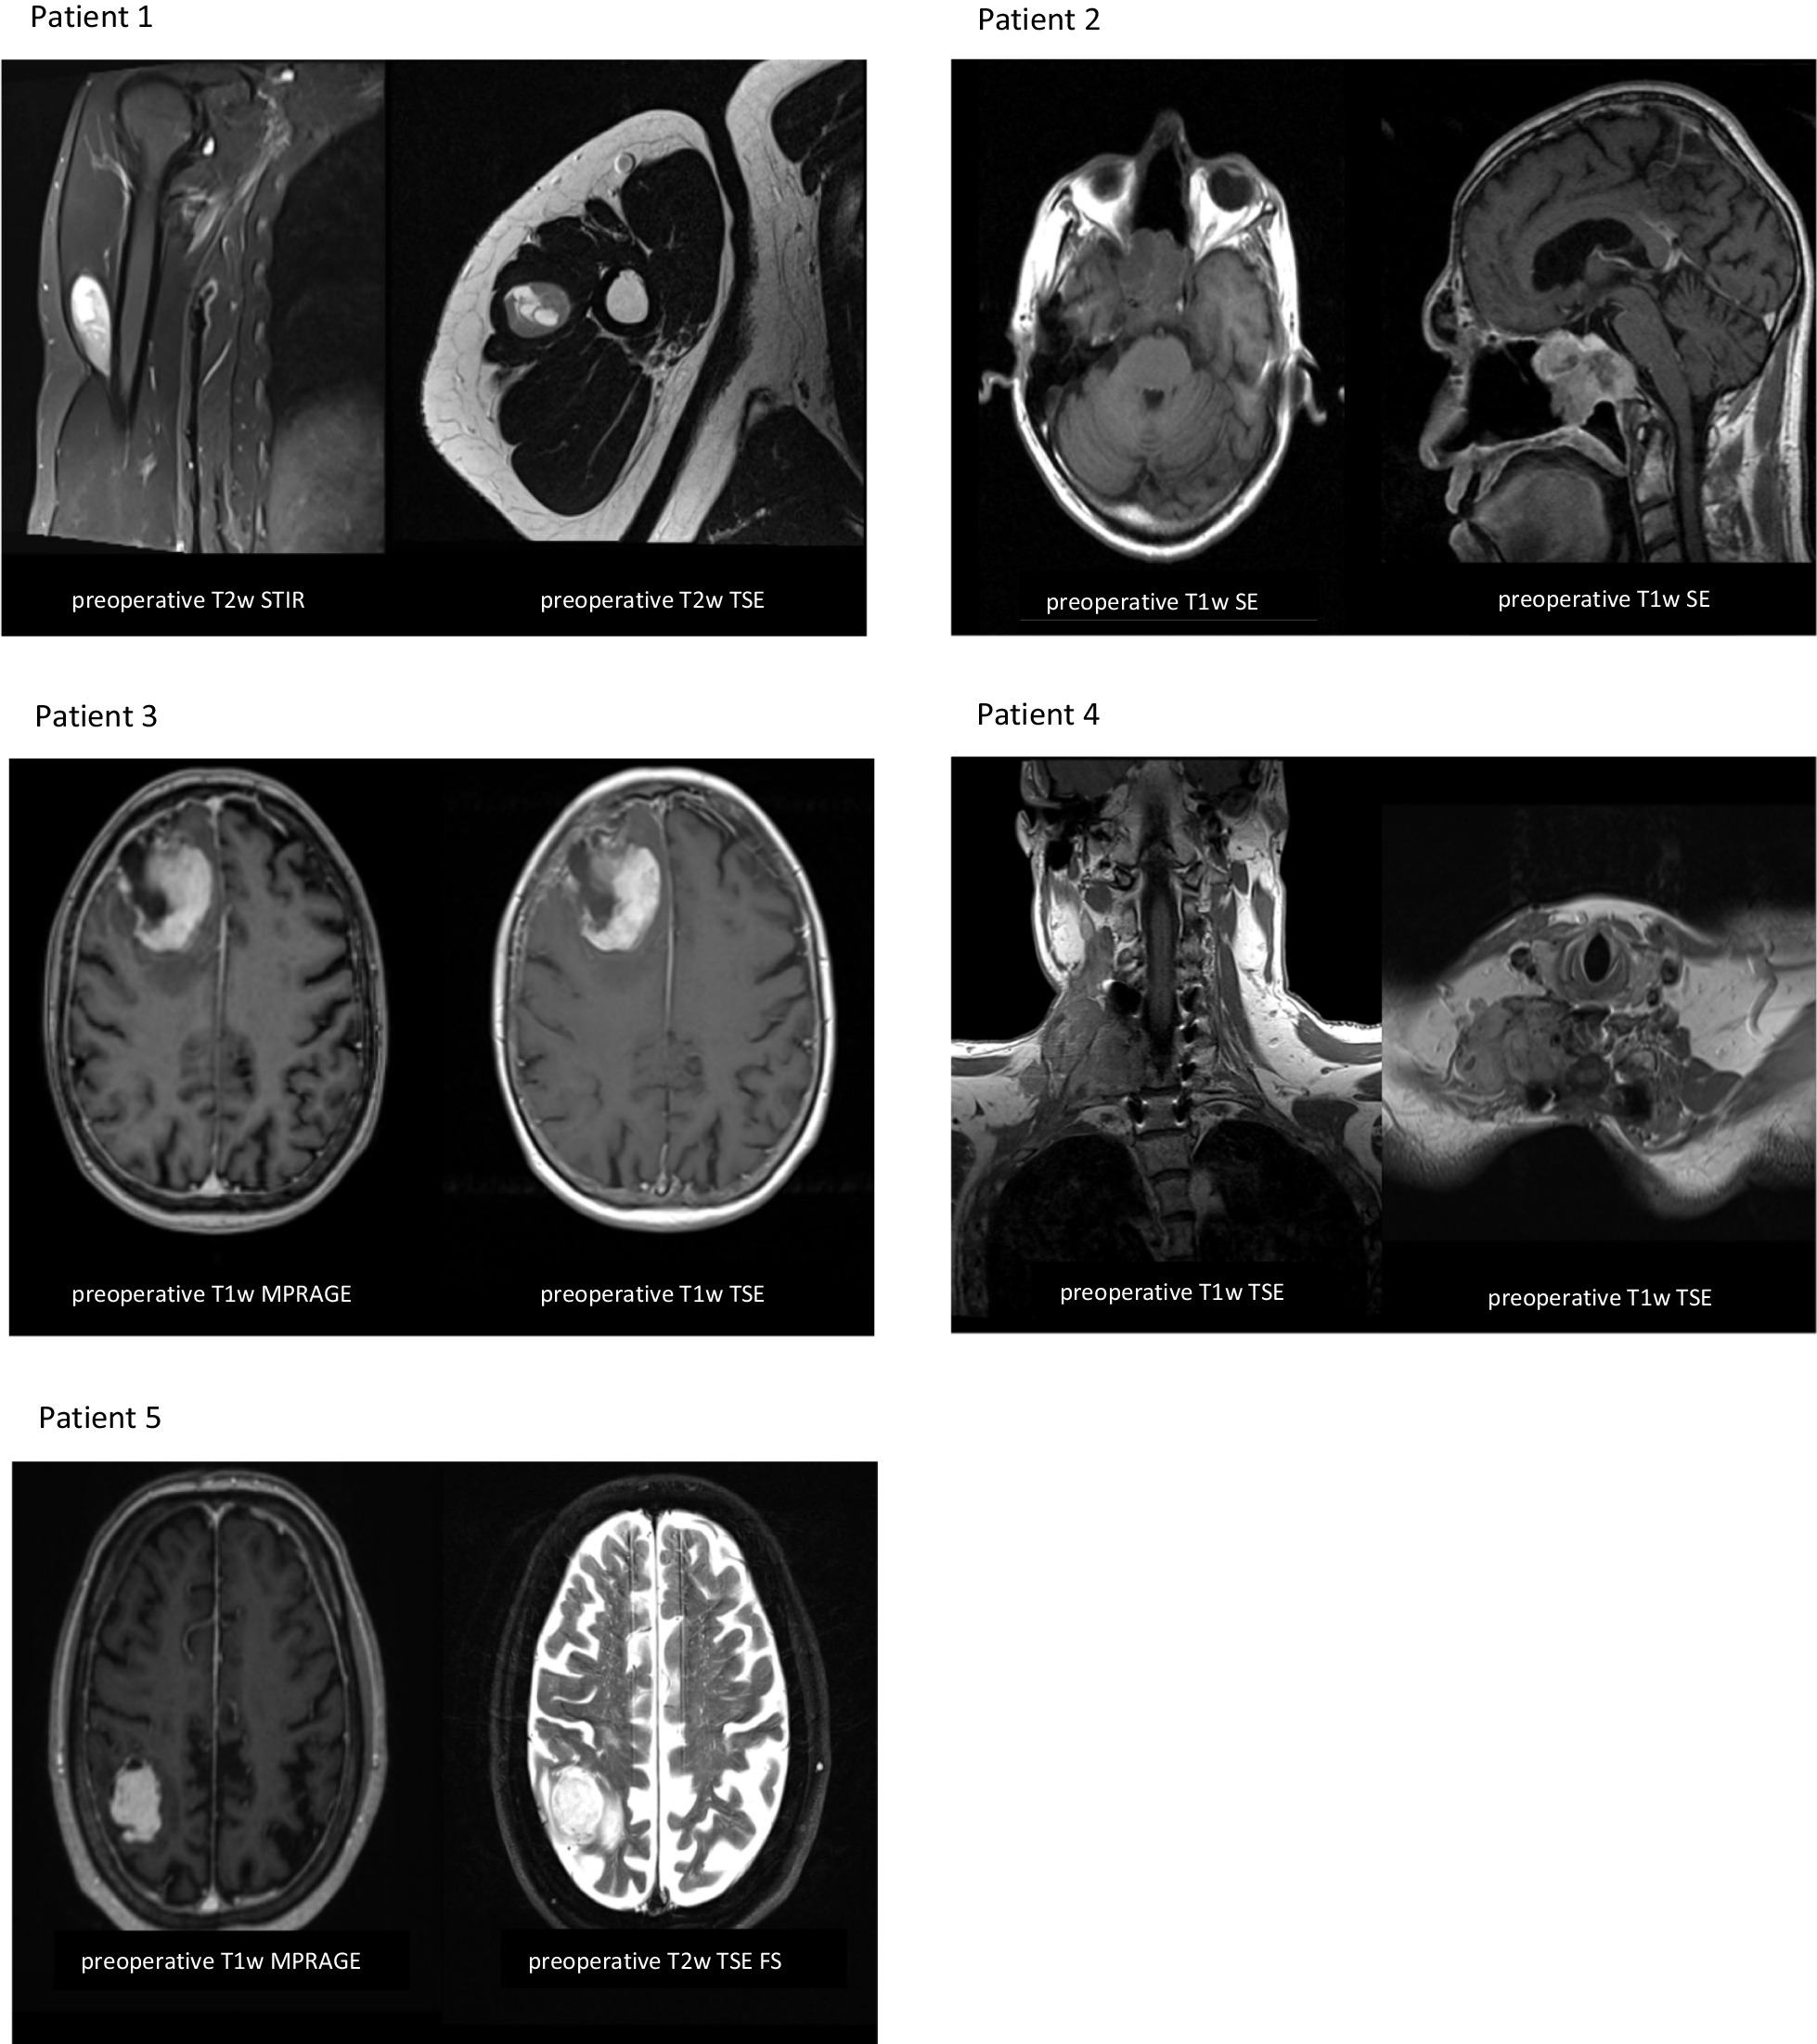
Preoperative MRI images. Representative MRI at the time of radiographic diagnosis and before treatment. *FS* fat saturation, *MPRAGE* magnetization prepared rapid gradient echo, *T1w* T1-weighted image, *T2w* T2-weighted image,* TSE* Turbo spin echo
